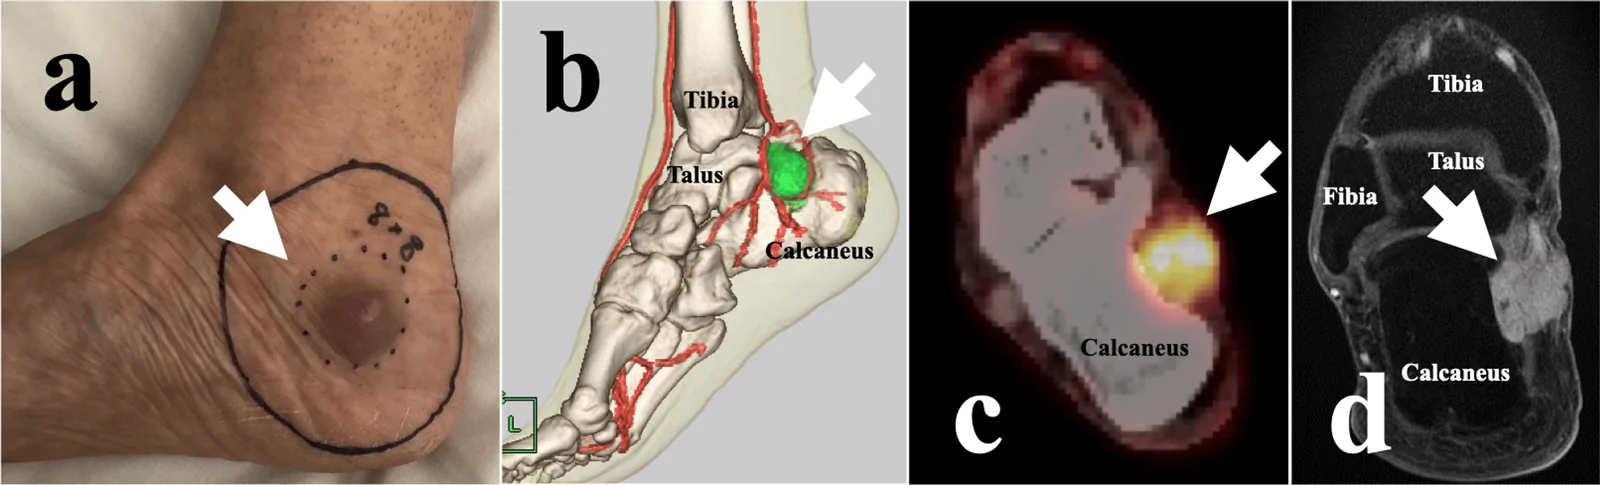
Preoperative lesion in the right heel (white arrows). (a) A photograph showing an elevated, circular, dark red lesion. (b) Sagittal 3D-CT showing the lesion above on the calcaneus. (c) Coronal PET/CT demonstrating intense FDG uptake in the lesion. (d) Coronal contrast-enhanced MRI demonstrating enhancement of the lesion. 3D, three-dimensional; CT, computed tomography; PET, positron emission tomography; FDG, 2-deoxy-2-[¹⁸F]fluoro-D-glucose; MRI, magnetic resonance imaging.
